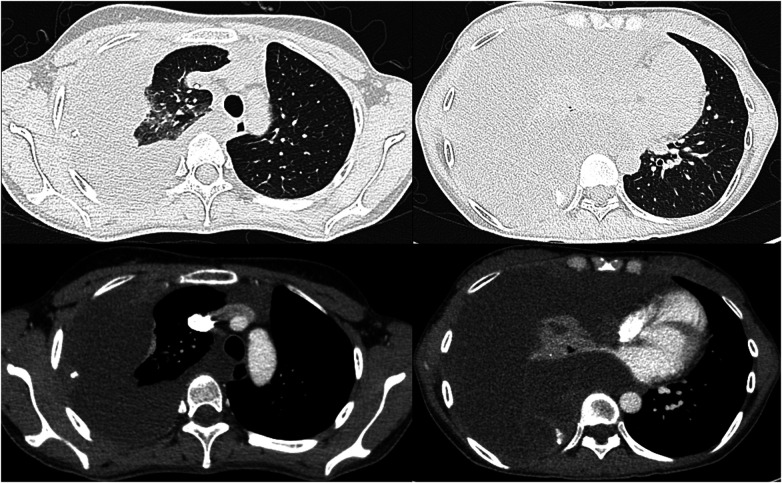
CT shows a large amount of relatively homogeneous fluid-density shadow in the right thoracic cavity, with multiple cystic lesions and patchy soft tissue-density shadows within.
